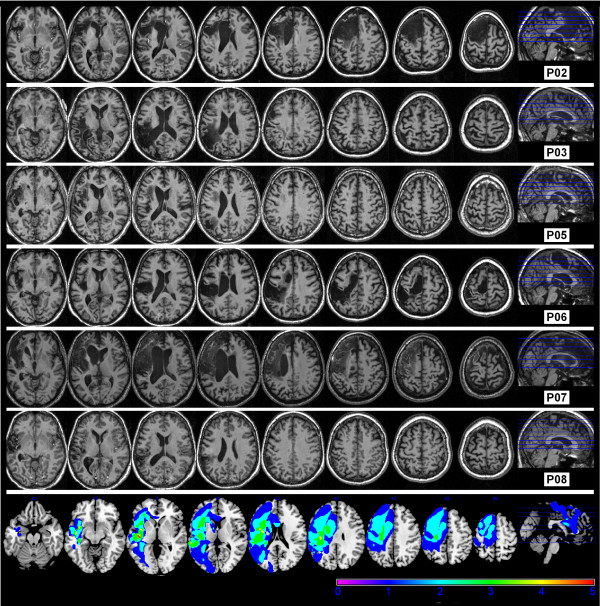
Lesion characteristics. Axial slices from a 3D T_1_ weighted MRI at 10 mm intervals for each participant with aphasia (starting at z = −6) and a lesion overlay map.
